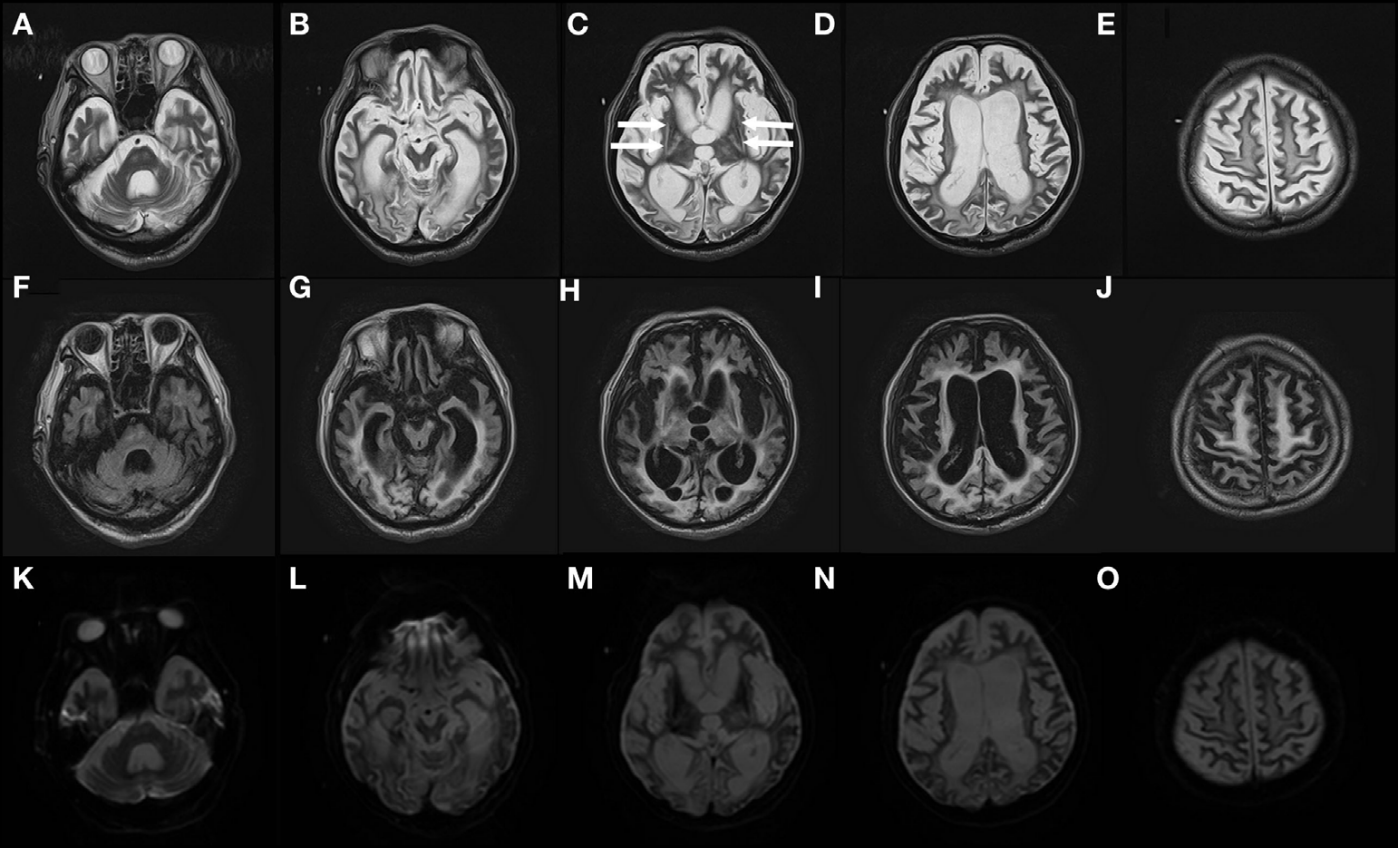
Severe atrophy in the MSA patient’s brain observed in August 2016 Gadolinium-enhanced MRI T2 images (TR: 4,800 ms, TE: 105 ms) (A–E), fluid-attenuated inversion recovery images (TR 10,000 ms, TE 120 ms) (F–J), and diffusion-weighted imaging images (TR 5,400 ms, TE 100 ms) (K–O) of the patient’s brain in August 2016 are shown. Arrows in (C) indicate dorsolateral regions of atrophy (“hyperintense lateral putaminal rim”). MSA, multiple system atrophy; MRI, magnetic resonance imaging.
